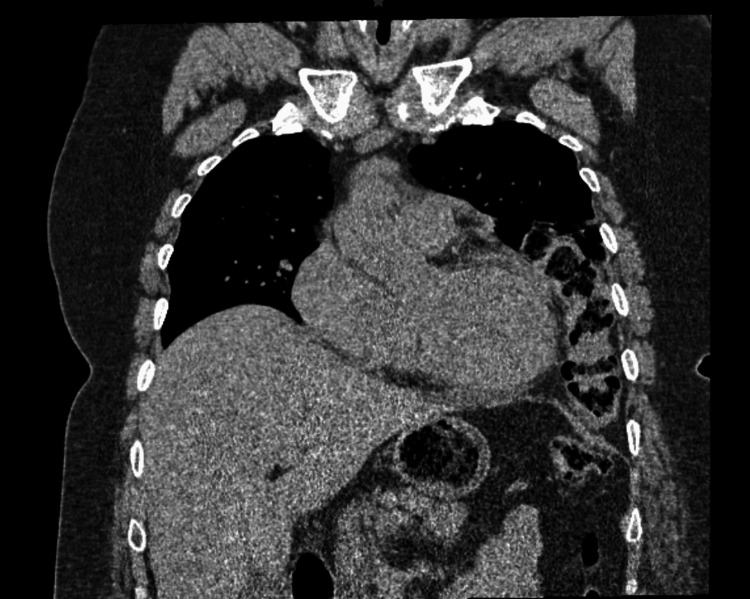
Coronal slice computed tomography (CT) scan: intrathoracic transverse colon. In this figure, the presence of abdominal content in the thoracic cavity can be identified, creating a mass effect and, therefore, restricting the left lung expansion.
